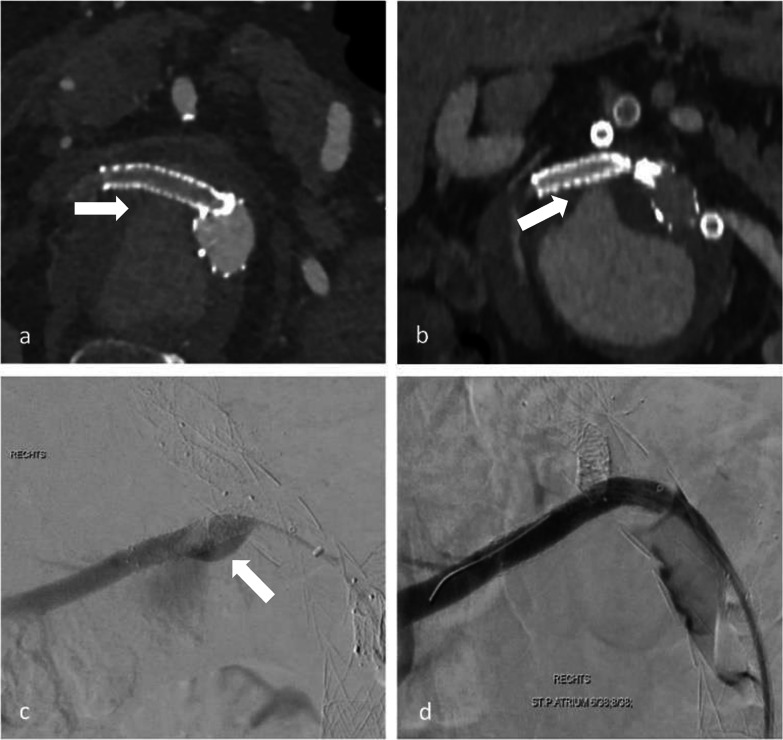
Detection of an unspecified endoleak directly adjacent to the right renal BSG in arterial and venous CTA (a, b), confirmed to originate from a membrane disruption via angiography (c) and after successful religning (d)
